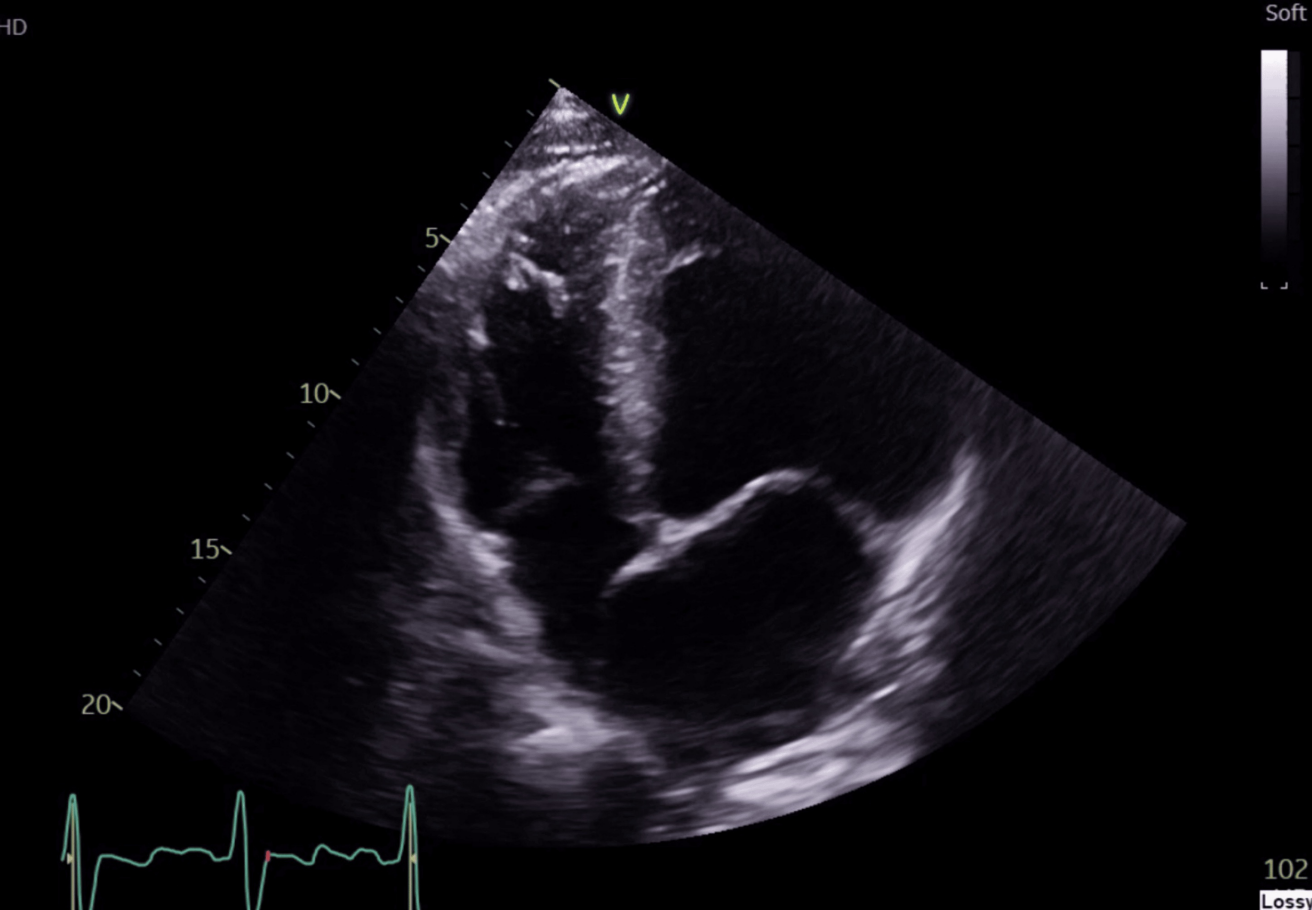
An apical four-chamber (A4C) view of the trans-thoracic echocardiogram, showing a dilated left ventricle cavity.
